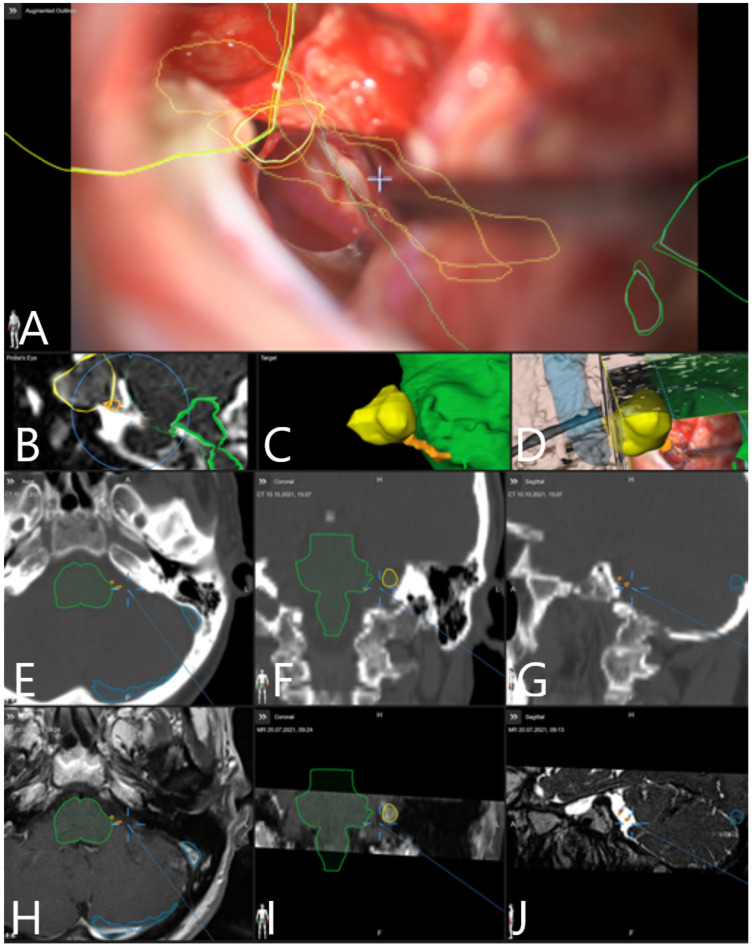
Patient No. 36, with left-sided AN and intact CN VII function. Patient underwent GTR of the tumor, and postoperative CN VII function was intact. (A) AR display on video screen with the outline of tumor (yellow), CN VII/VIII complex origin with course of CN VII at brain stem (orange) and brain stem (green). (B) Corresponding probe’s eye view. (C) Target view (tumor and further objects outside of the focus plane are visualized) and (D) overview video plane in relation to the 3D objects. (E) Navigation display with focus plane on the CN VII/VIII course with segmented objects (tumor outline in yellow, CN VII/VIII in orange, venous sinuses in blue) following craniotomy within CT mode in (E) axial, (F) coronar and (G) sagittal view as well as in (H) axial and (I) coronar T1-weighted post-contrast MRI and (J) sagittal view of T2-weighted MRI.
